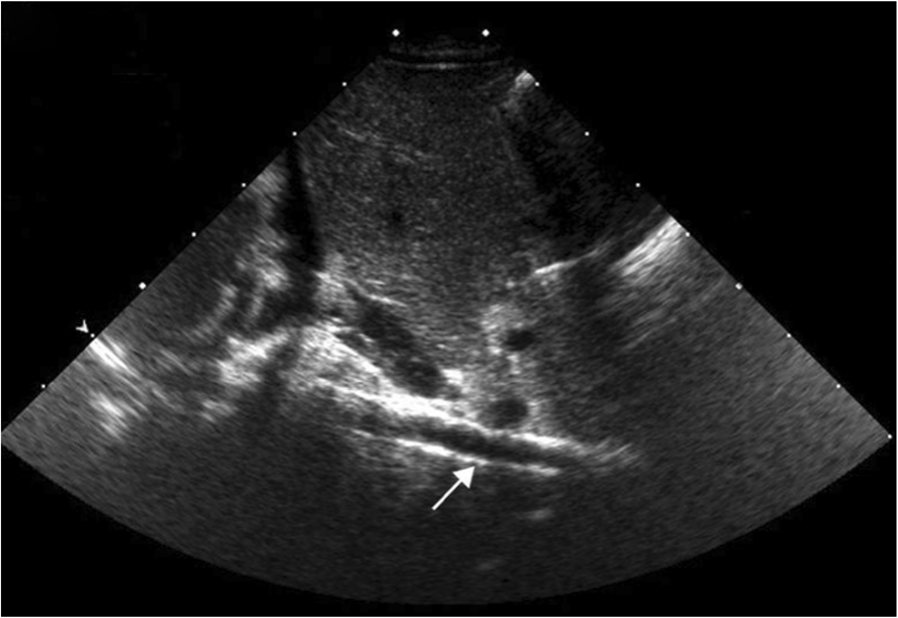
Ultrasound of abdomen showing calcified aorta.
